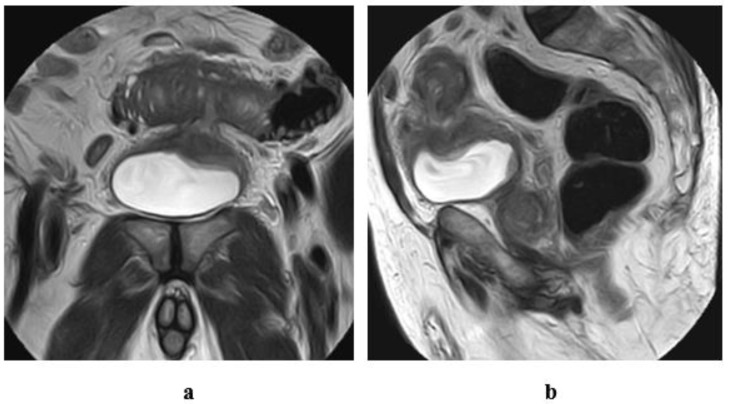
A case of eosinophilic cystitis secondary to intestinal diverticulitis and subsequent vesicointestinal fistulous communication, in a 77-year-old male patient. T2 w MRI of the pelvis (a) coronal, (b) sagittal views: localized thickening of and a defect at the dome of the bladder, associated with track formation extending from the dome to the bowel loop superior to the bladder. Inflammatory reaction and diverticulitis of the related portion of the intestinal loop.
